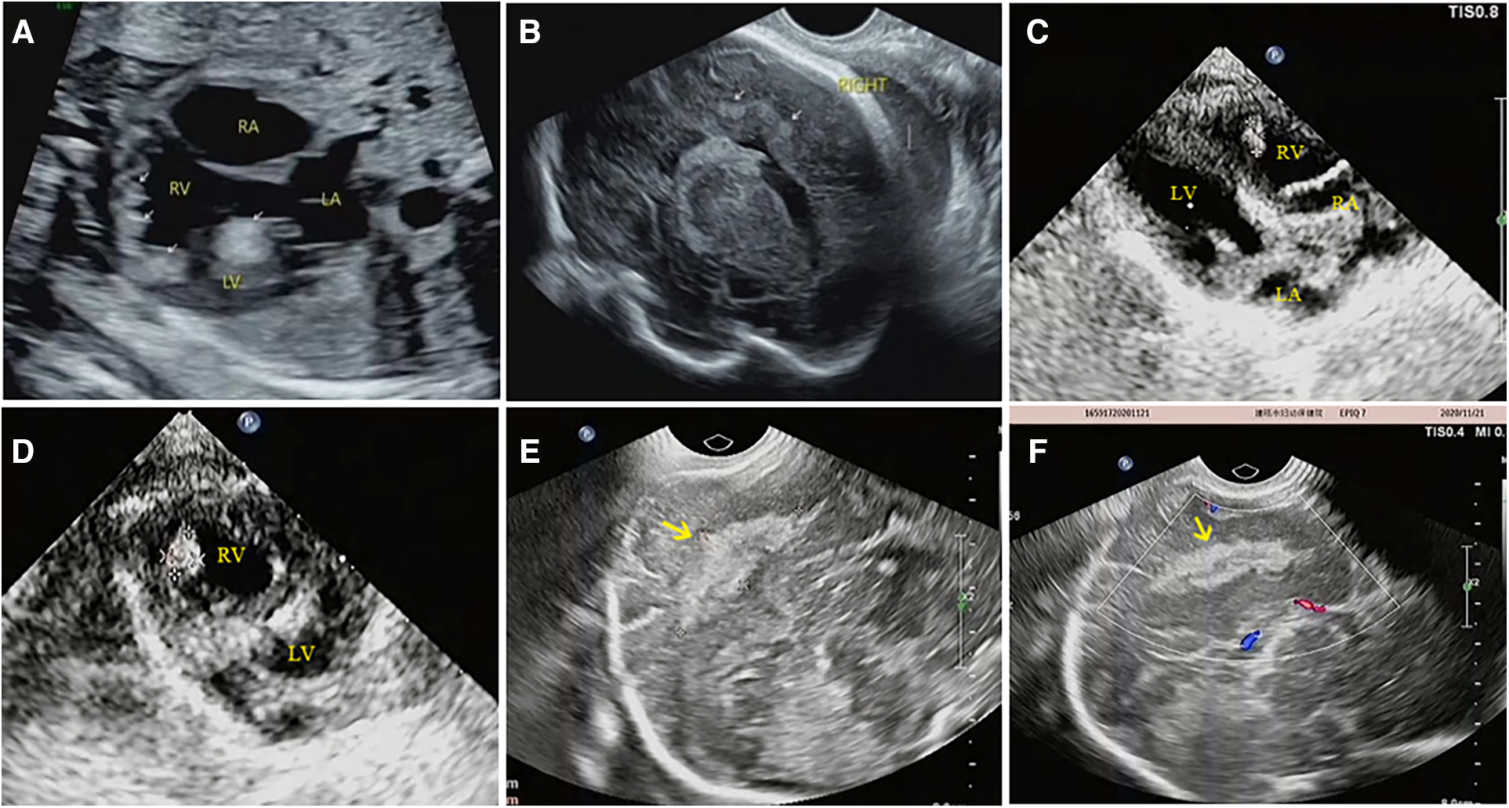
Prenatal and postnatal ultrasonography of cardiac rhabdomyoma complicated with cranial sclerotic nodules in case NO. 13. (A) Multiple slightly hyperechoic nodules in the ventricle of the fetal heart at 28 weeks of gestation. (B) Multiple hyperechoic intracranial nodules of the fetal heart at 28 weeks of gestation; however, no intracranial lesions were detected by MRI at the same time. (C,D) Multiple slightly hyperechoic nodules in the ventricle of the baby at 1 month after birth. (E,F) Hyperechoic intracranial nodule of this baby at the same time.
